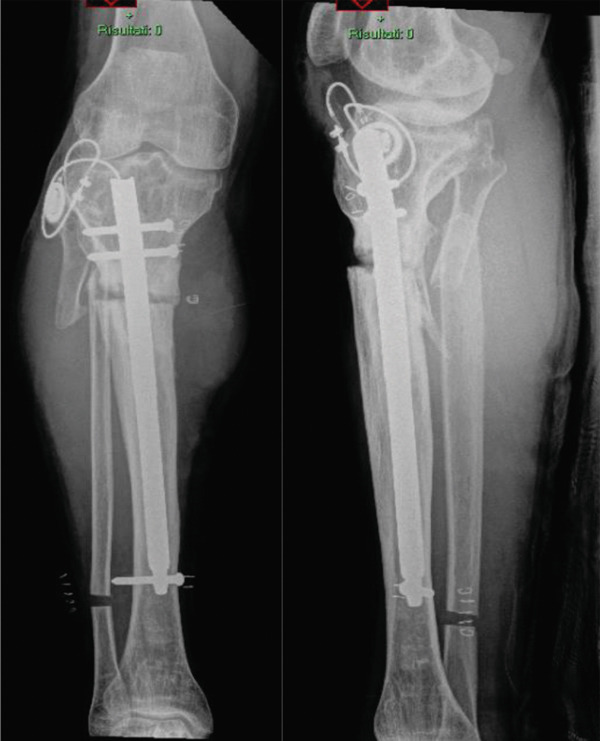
Radiograph showing the position of intramedullary lengthening nail with tibial and fibular lengthening osteotomy.
